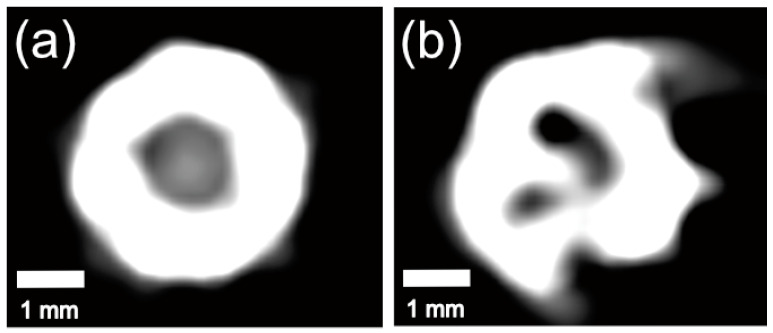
Axial slice of a stent (Boston Scientific Promus Element (Boston Scientific Corporation, Marlborough, MA, USA)) at rest (a) and in motion (b). PCD CT in UHR mode using the Bv60 kernel. The window width was set to 1500 HU and the center to 300 HU.
